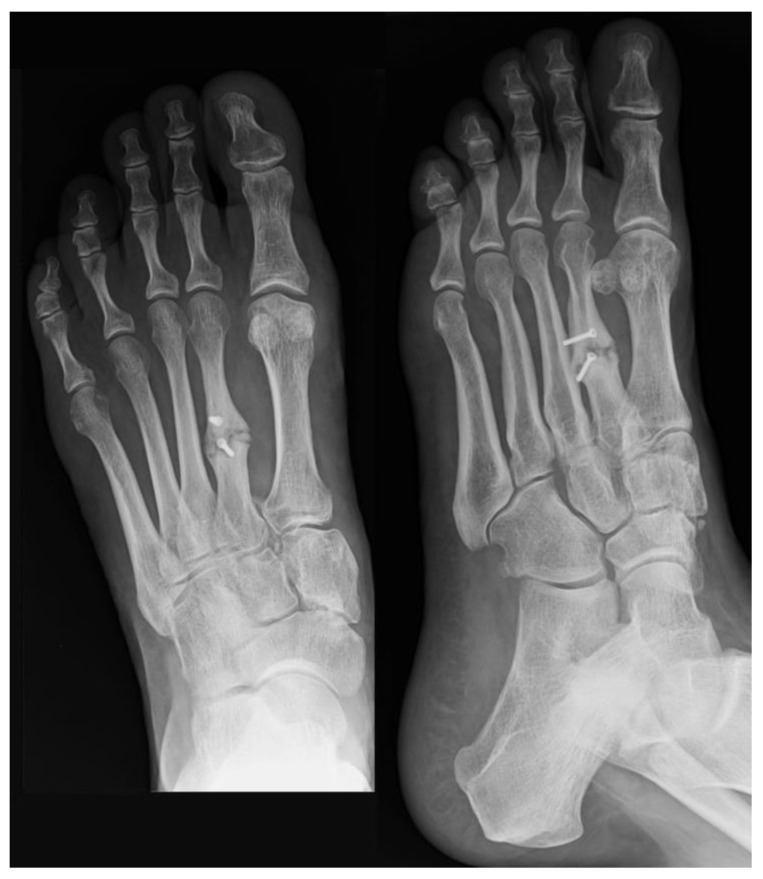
Weight-bearing dorsoplantar X-rays of the left foot revealing nonunion of the second metatarsal and a mild alteration in the metatarsal formula.
